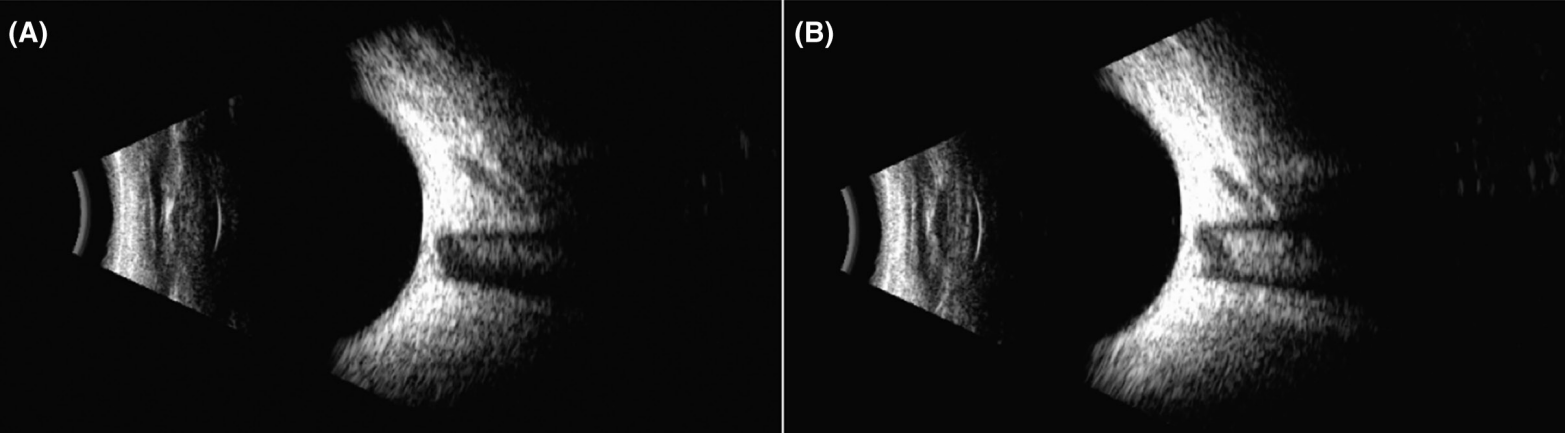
B‐scan ultrasonography of the left eye (LE) (A) and the right eye (RE) (B) revealed no characteristic changes.
